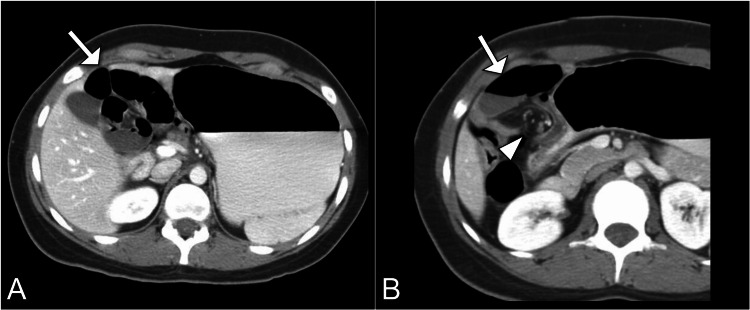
Axial CT images reveal a cluster of dilated jejunal loops (arrow) in the right upper quadrant (A) and a twist in the blood vessels (arrowhead) (B), indicative of a right paraduodenal hernia. CT, computed tomography
